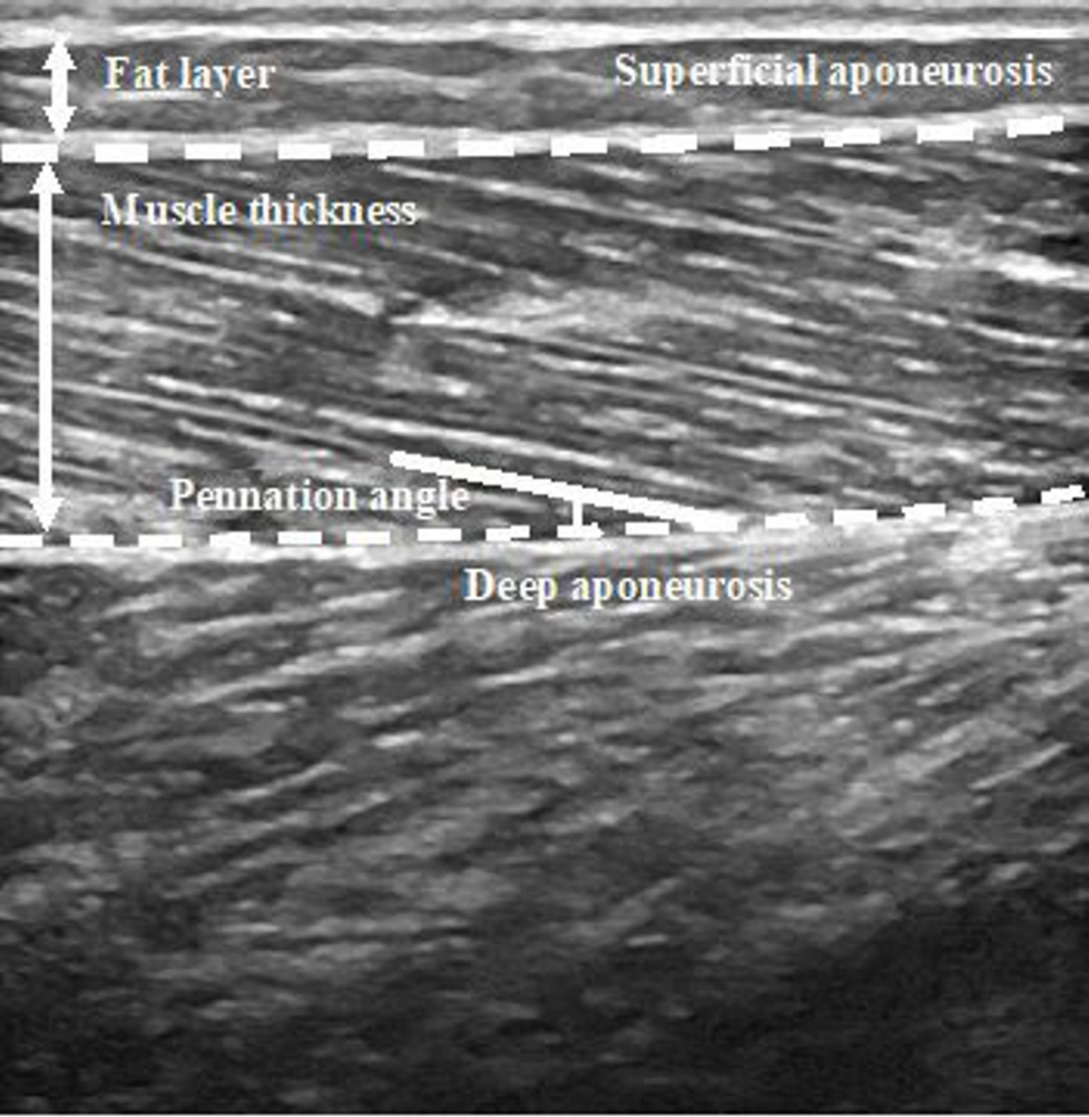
Example of fascicle tracking on an ultrasound image. Muscle thickness was calculated as the distance between the deep and superficial aponeurosis. Pennation angle was calculated as the angle between the deep aponeurosis and the tracked fascicle.
